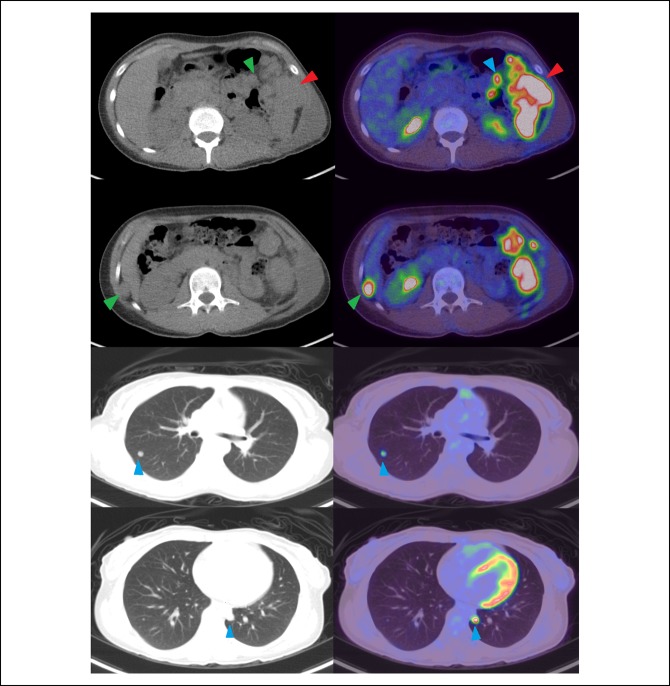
CT (left) and fused (right) FDG-PET CT images showing the large irregular left abdominal wall soft-tissue mass with intense FDG-uptake (red arrowheads), peritoneal lesions (green arrowheads), and FDG-avid bilateral pulmonary nodules (blue arrowheads).
